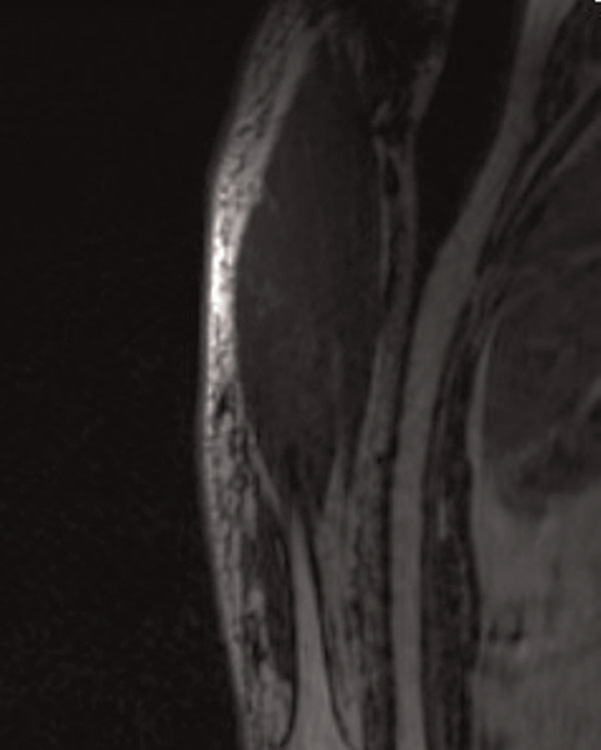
Magnetic resonance imaging demonstrating showed fibrotic infiltrate from radiotherapy.
